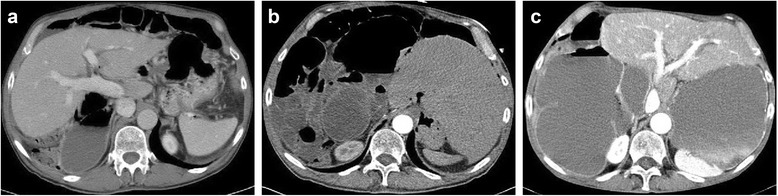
Transverse CT scans demonstrating the liver to be located to the left and right of the midline on different occasions. a Year 2009. b Year 2012. c Year 2014
